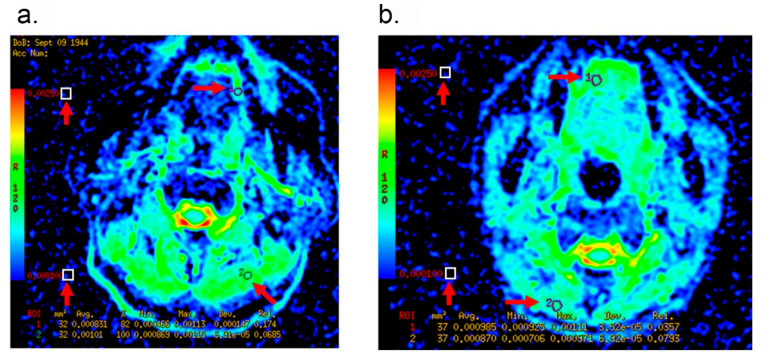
Representative MRI images of patients with cT1-2 OTSCC. Arrows indicate the region of interest (ROI) of the tumor and the non-tumor tissues for ADC measurements. (a): A 70-year-old male patient with Recurrence. The ADC value of tumor was measured as 0.831 × 10^−3^ mm^2^/s, and the ADC ratio was 0.822. (b): A 41-year-old female patient without Recurrence. The ADC value of tumor was measured as 0.985 × 10^−3^ mm^2^/s, and the ADC ratio was 1.132.
